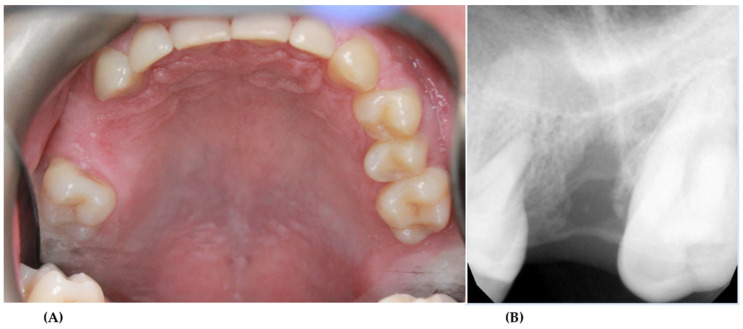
Intraoral photographs after 10th mouths after surgical treatment (A); dental X-ray—10th months after 27 extraction—stabilization of bone healing (B).
